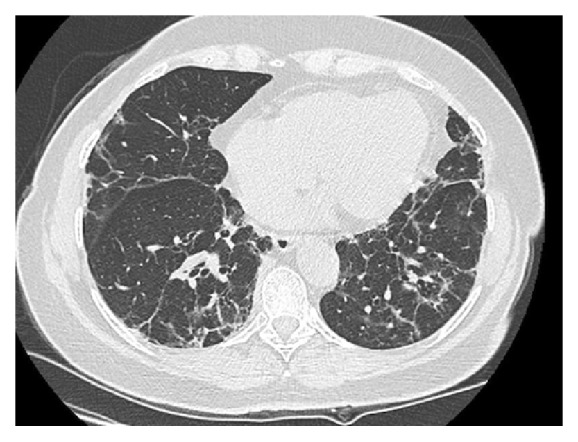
The chest CT scan showing the persistence of a bilateral interstitial syndrome associated with bronchial dilatation and pleural-based consolidations 1 year after ARDS.
